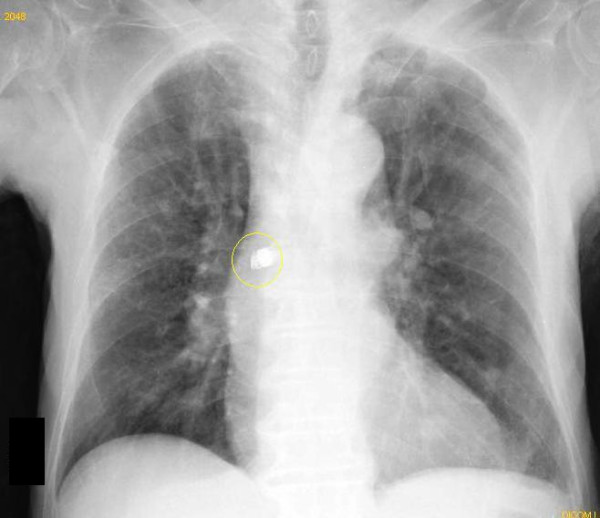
Second X-ray of the chest. Two days later, another X-ray of the chest was performed showing the capsule in the right bronchus.
